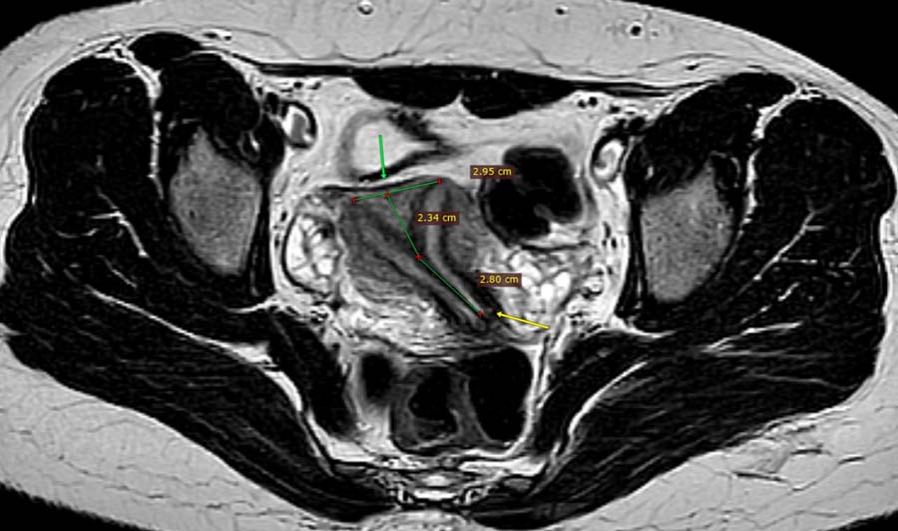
MRI pelvis, axial T2, complete separate uterine cavity (complete septate uterus), two cervices openings (below arrow), no fundal cleft, inter-cornual distance = 29.5 mm (above arrow), the upper 23.5 mm of the septum is composed of myometrium, while the distal 28 mm is composed of fibrous tissue.
